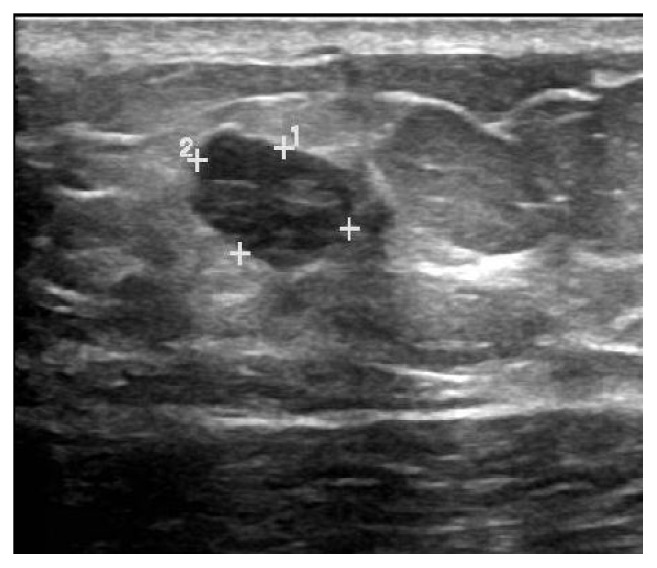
Breast ultrasound: lobulated solid left breast mass at 11:00, approximately 0.8 × 1.1 × 1.4 cm.
